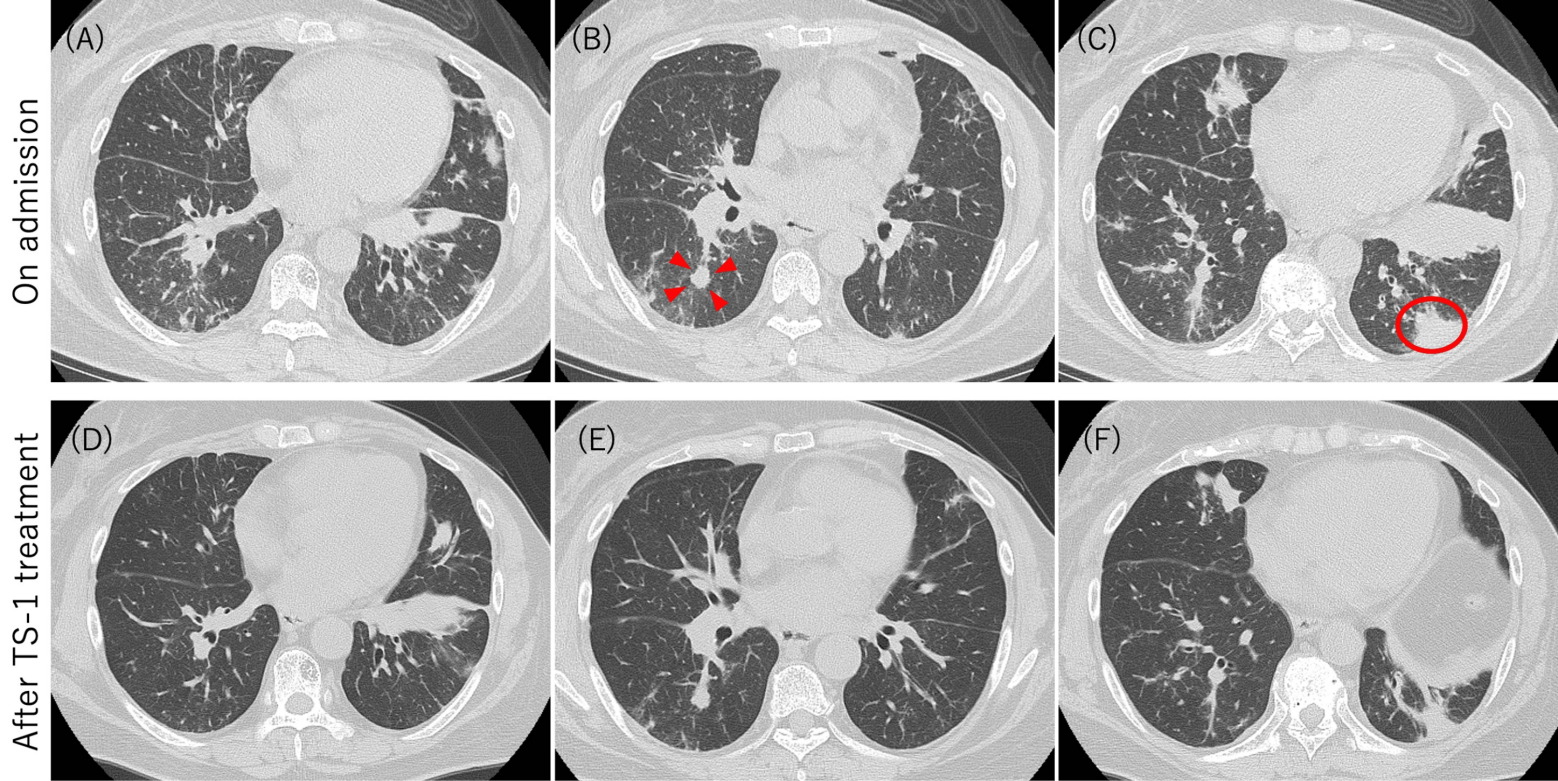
Serial chest CT findings on admission and after S-1 therapy Axial thin-section CT images obtained on admission (A-C) demonstrate multifocal OP-like air-space opacities, including patchy peribronchovascular and subpleural consolidation with surrounding ground-glass changes. Arrowheads in B indicate preexisting metastatic lesions recognized prior to admission. The circled area in C marks the target lesion from which the transbronchial biopsy was performed. Follow-up CT images after S-1 treatment (D-F), at corresponding levels, show marked interval regression of the consolidations and metastatic opacities, with only minimal residual linear or reticular changes. OP, organizing pneumonia
